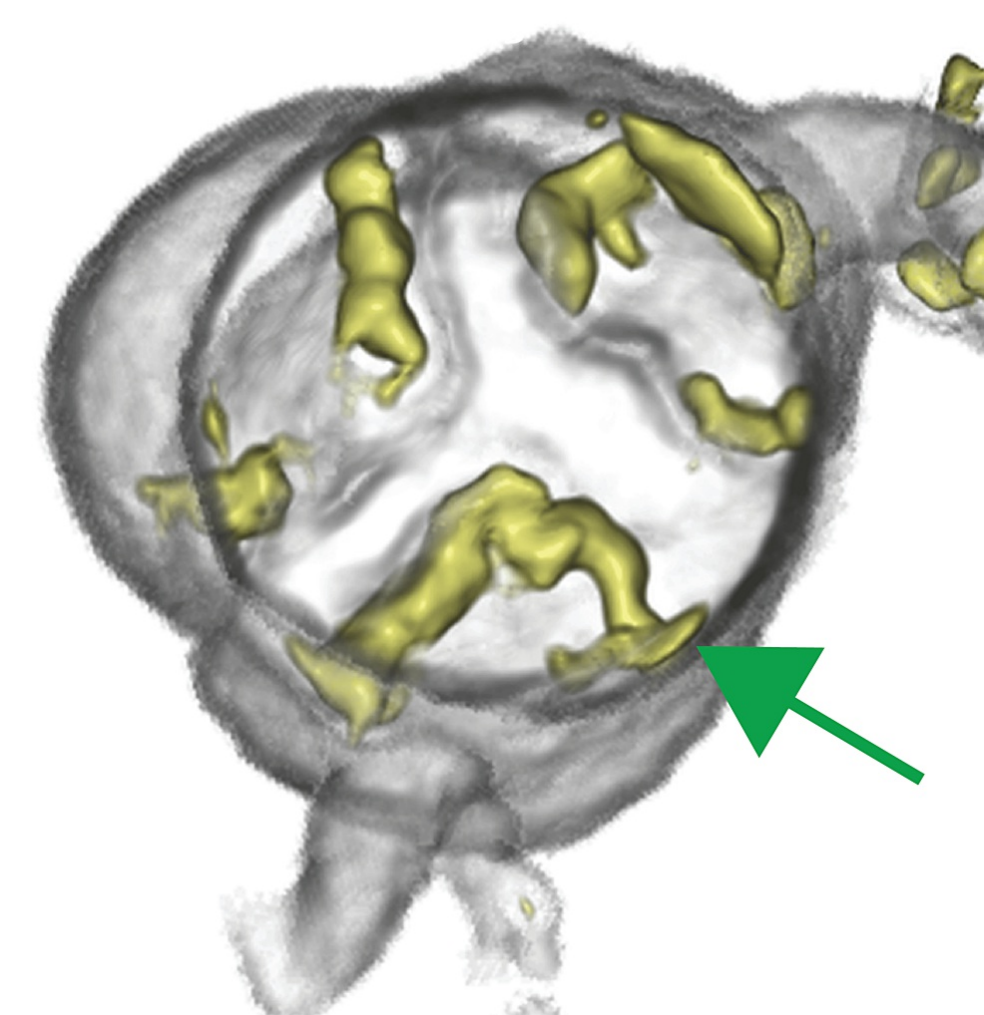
Preoperative images of 3D enhanced computed tomography The aortic valve was observed from the cephalic side. This figure demonstrates the preoperative state of the aortic valve with tricuspid morphology and severity (green arrow).
